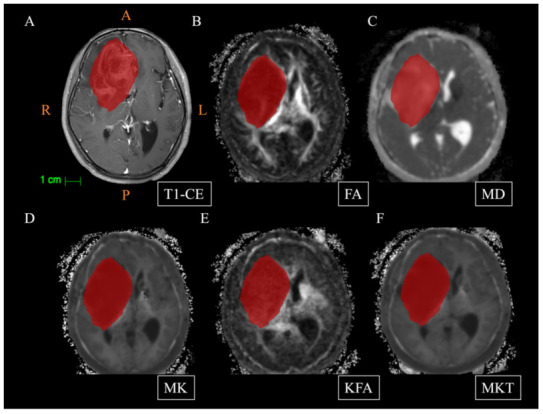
Delineation of volumes of interest (VOIs). A 60-year-old man with glioblastoma (WHO grade IV). (A): The lesion shows significant inhomogeneous enhancement on axial T1-weighted enhancement imaging. (B–F): fractional anisotropy (FA) parameter maps, mean diffusivity (MD) parameter maps, mean kurtosis (MK) parameter maps, kurtosis fractional anisotropy (KFA) parameter maps, and mean kurtosis tensor (MKT) parameter maps, respectively (the VOIs are outlined in red on each parameter image). A: Anterior; P: Posterior; R: Right; L: Left.
